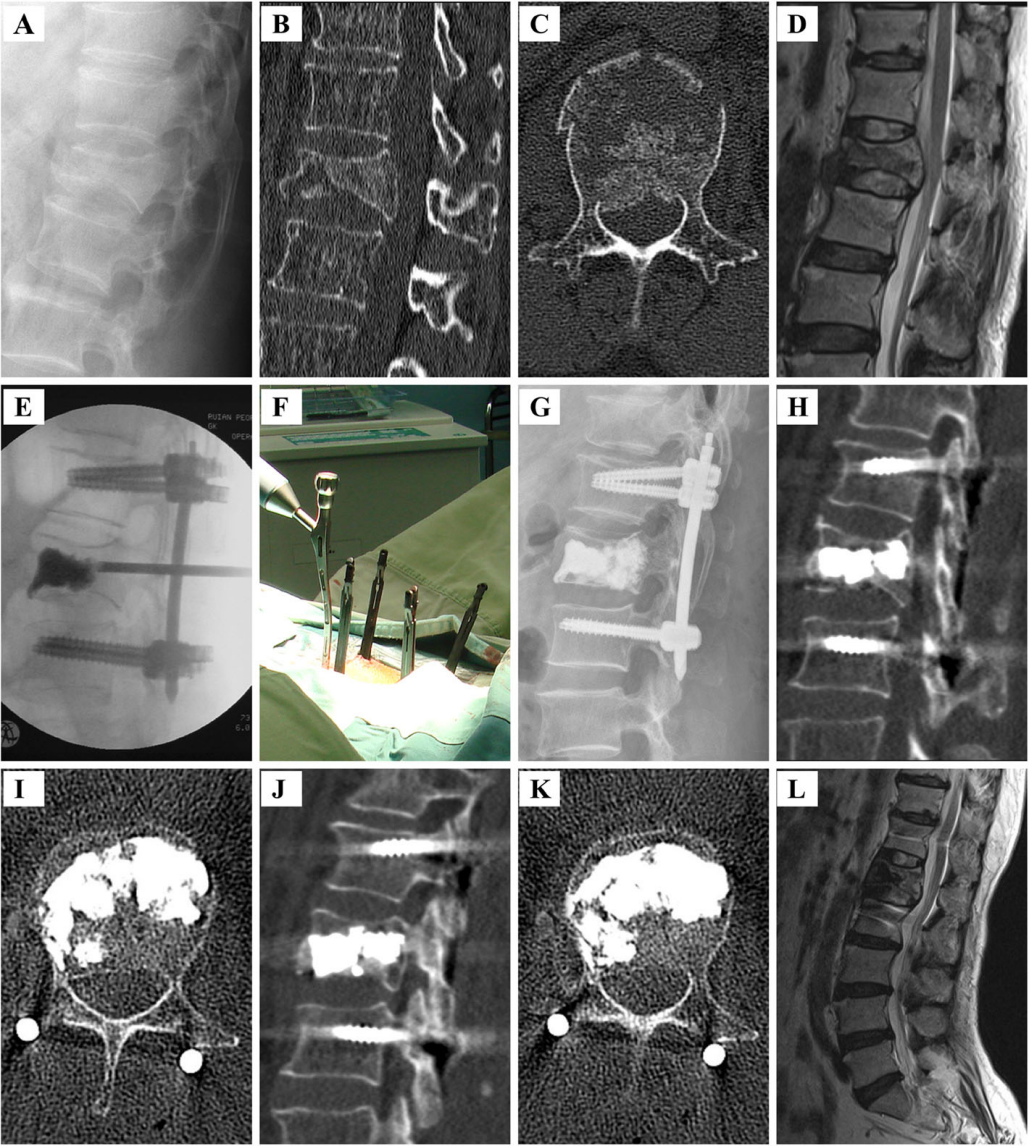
Female patient of 60 years with OVBFs in L_1_ vertebra undergoing pedicle screw fixation plus vertebroplasty. A Preoperative lateral radiograph showing a burst fracture of L_1_. B, C Preoperative CT-scan (plain and sagittal reconstruction image) showing the burst fracture with spinal canal compromise. D Preoperative MRI (T2-weighted sequences) showing the burst fracture with spinal canal compromise. E Intraoperative fluoroscopic image demonstrating percutaneous pedicle screws in the adjacent vertebrae and vertebroplasty in the fractured vertebra. F Intraoperative view. G Postoperative lateral roentgenogram showing adequate vertebral body reduction, excellent alignment, and reduced spinal canal encroachment following short fixation and adequate vertebral body reduction. H, I CT-scan (plain and sagittal reconstruction image) showing adequate vertebral body reduction, excellent alignment and reduced spinal canal encroachment following short fixation and adequate vertebral body reduction. J, K CT-scan (plain and sagittal reconstruction image) of the patient 1 year after surgery showing vertebral body reduction, alignment and reduced spinal canal encroachment without significant loss of correction. L T2-weighted sagittal MRI of the patient 4 years after surgery showing reduced spinal canal encroachment and excellent alignment and adequate vertebral body reduction
